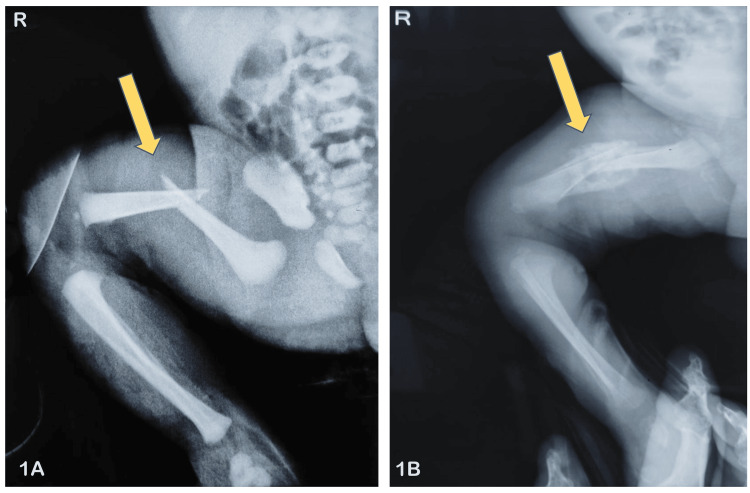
X-ray of the right femur (1A) one day after childbirth and (1B) three weeks after treatment Area of fracture marked with a yellow arrow
